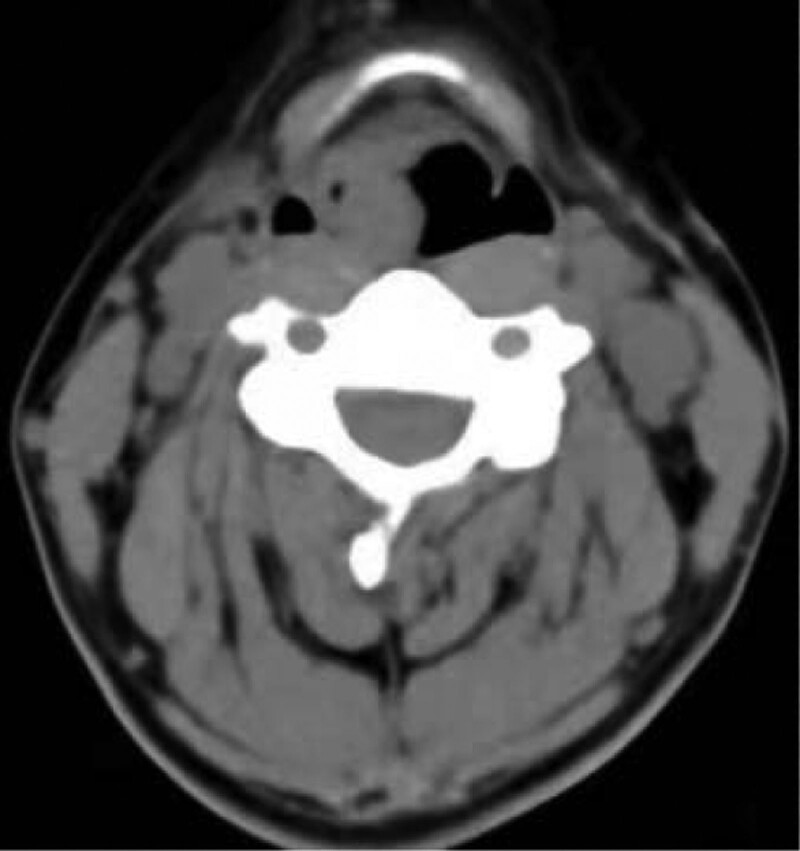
The CT imaging data of the patient. CT = computed tomography.
